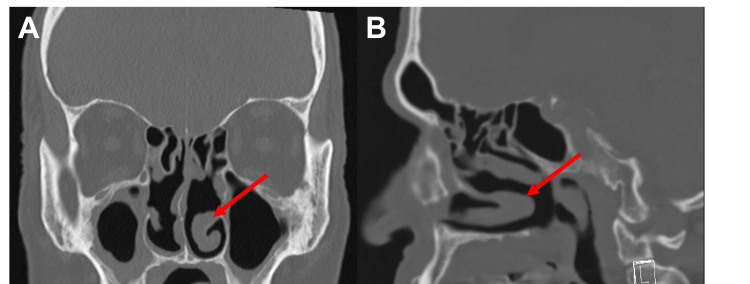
CT sinus revealing chronic sinusitis Chronic sinusitis and postsurgical changes with no findings to indicate superimposed acute sinusitis. Mucosal thickening bilaterally in the frontoethmoidal recesses is noted.
